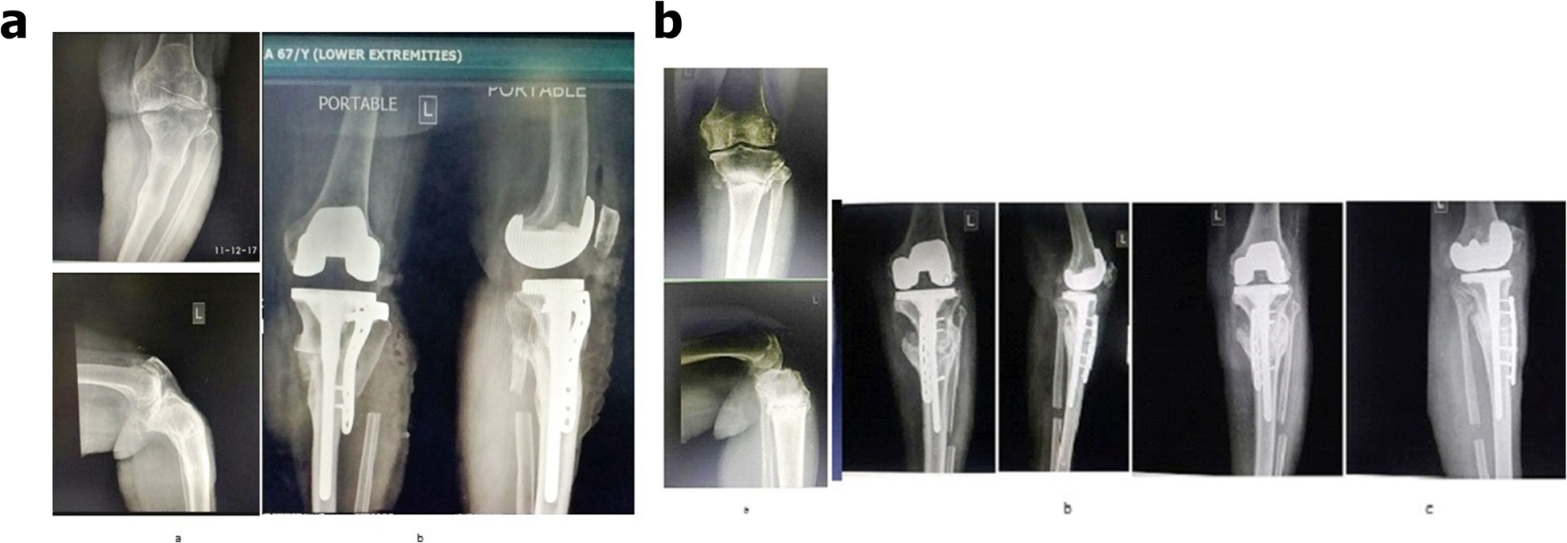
a: Left Knee X-rays. a) Preoperative anteroposterior and lateral view showing features suggestive of osteoarthritis, with deformity with stress fracture of proximal tibia; b) Recent follow-up anteroposterior and lateral view showing healed stress fracture with correction of deformity with modular stemmed knee prosthesis with implant (plate) in situ with proximal fibular resection. b: Left Knee X-rays. a) Preoperative anteroposterior and lateral view showing features suggestive of osteoarthritis, with deformity with stress fracture proximal tibia; b) follow-up anteroposterior and lateral view showing healed stress fracture with correction of deformity with modular stemmed knee prosthesis with implant (plate) in situ with proximal fibular resection; c) Recent follow-up anteroposterior and lateral view showing healed stress fracture with correction of deformity with modular stemmed knee prosthesis with implant (plate) in situ with proximal fibular resection
